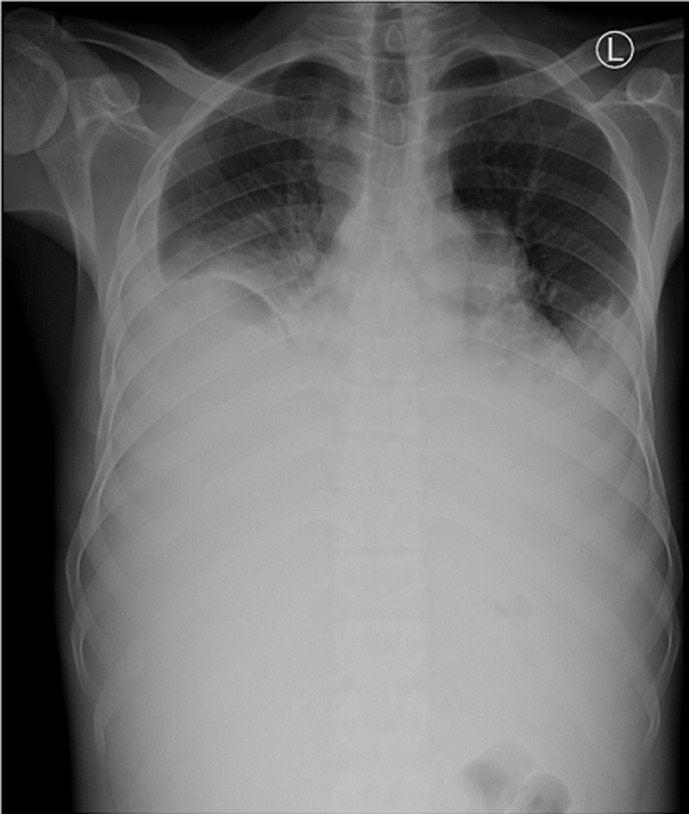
chest X-ray on admission showing massive bilateral pleural effusion
